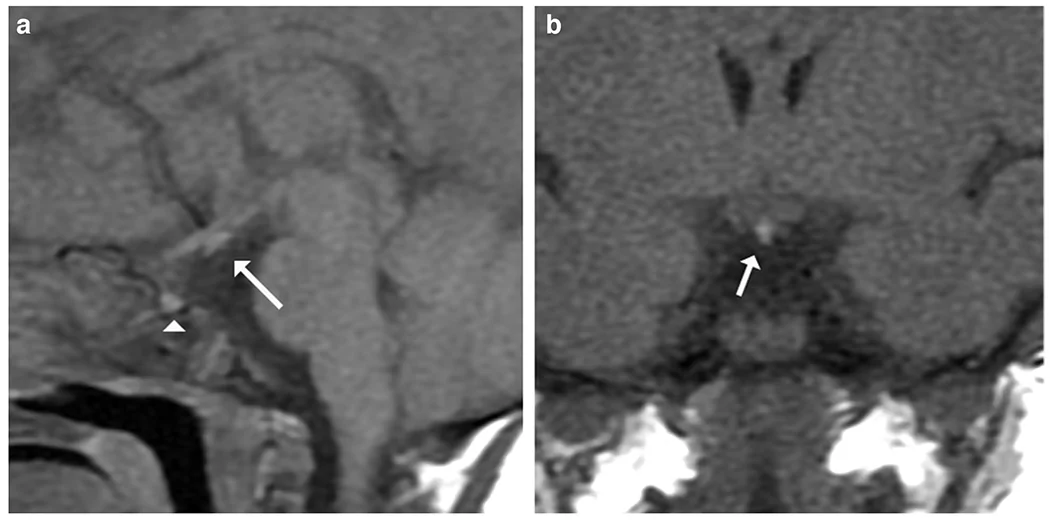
One-month-old female infant with multiple pituitary hormone deficiencies and pituitary stalk interruption syndrome. a Sagittal and (b) coronal T1-weighted images through the hypothalamus and sella show an ectopic posterior pituitary positioned at the median eminence of the hypothalamus (*arrow*), absence of the pituitary stalk, and hypoplasia of the adenohypophysis (*arrowhead*)
